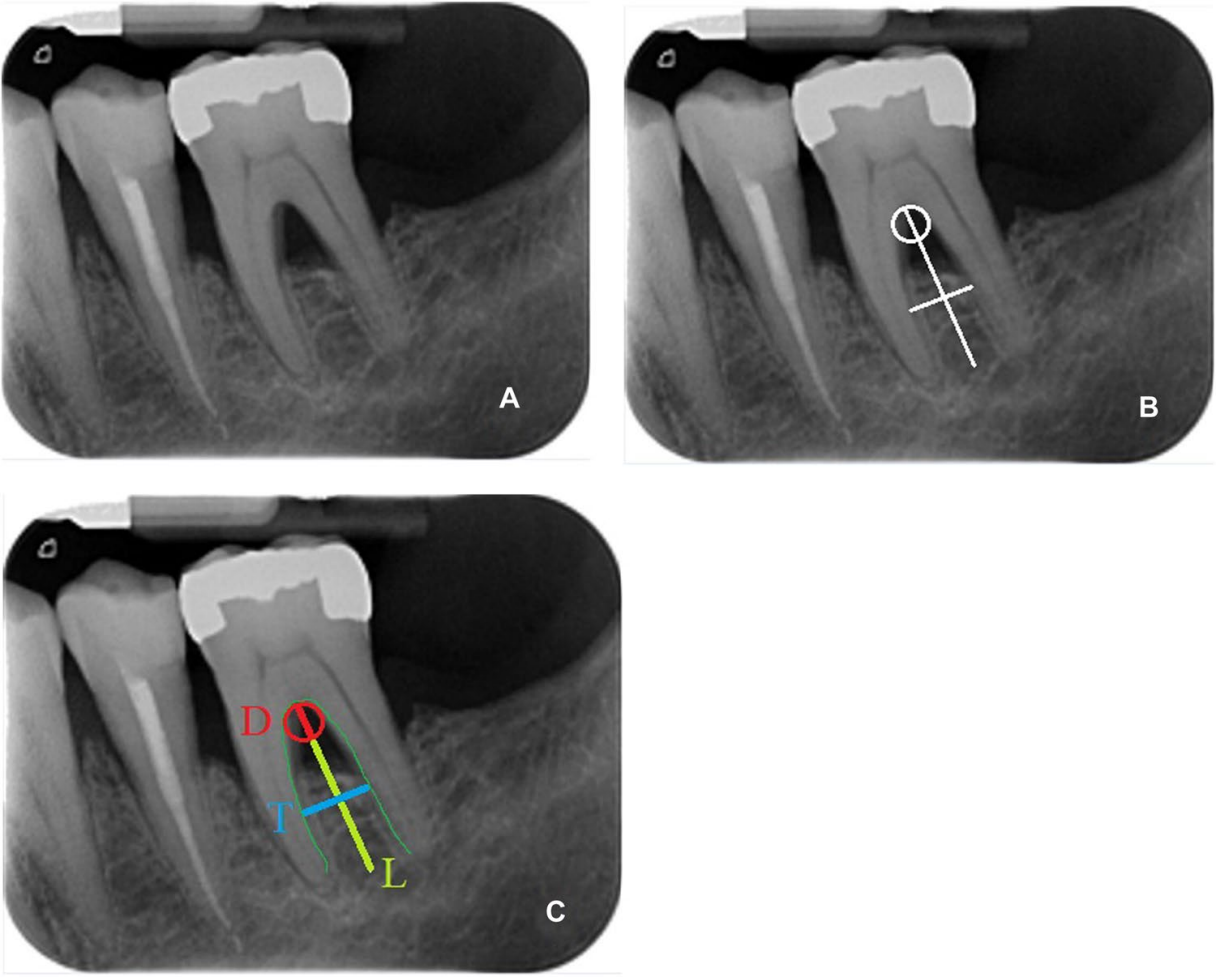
A A peri-apical X-ray lower molar. B The visual evaluation method. The osculating circle, the trasversal width and the longitudinal length are drawn on the rx. C Diameter of the osculating circle (*D*, in red); trasversal width (*T*, in light blue); longitudinal length (*L*, in yellow)
